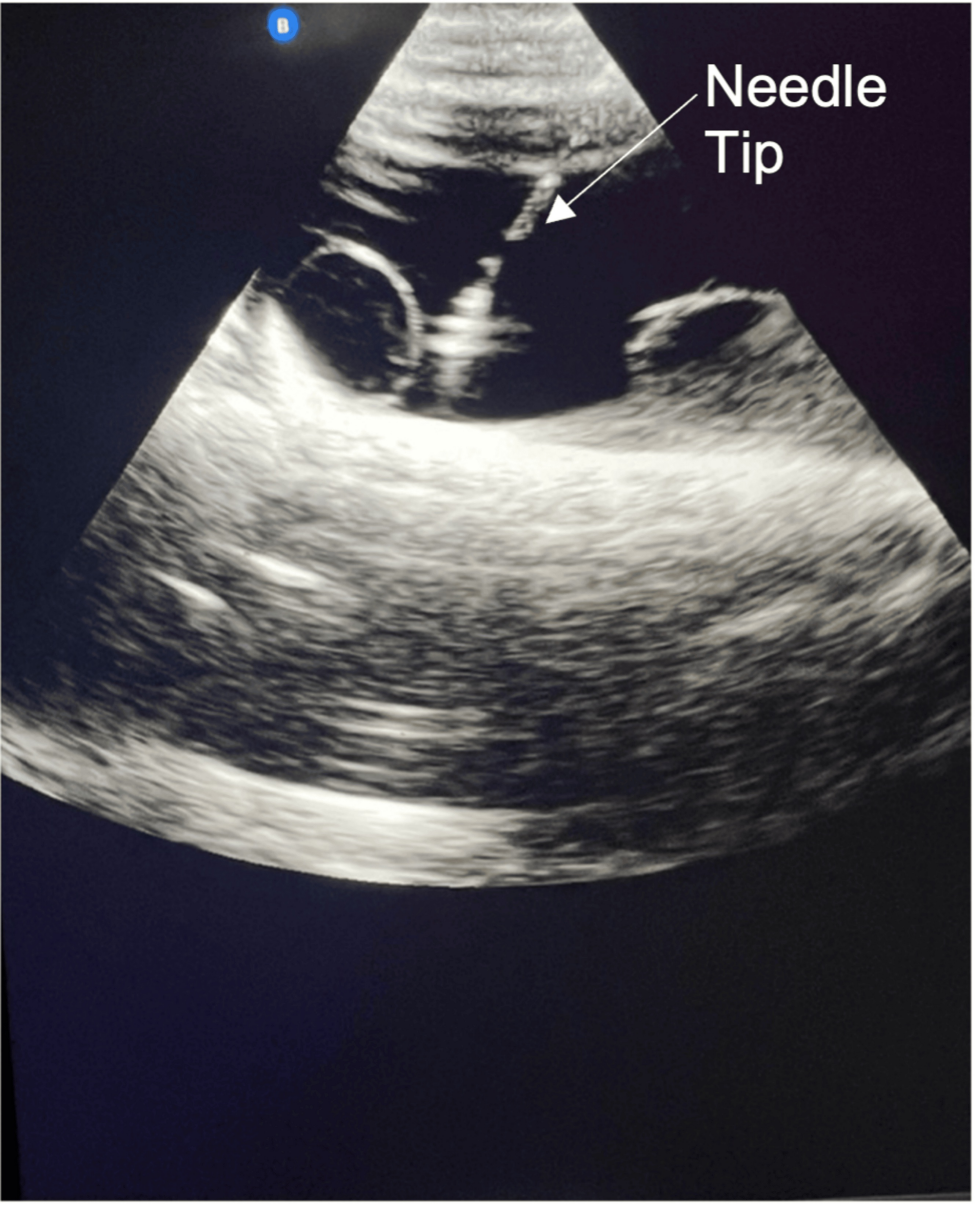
USGAP model with suprapubic catheter being guided into the ascitic fluid pocked using real time ultrasound USGAP: ultrasound-guided abdominal paracentesis
